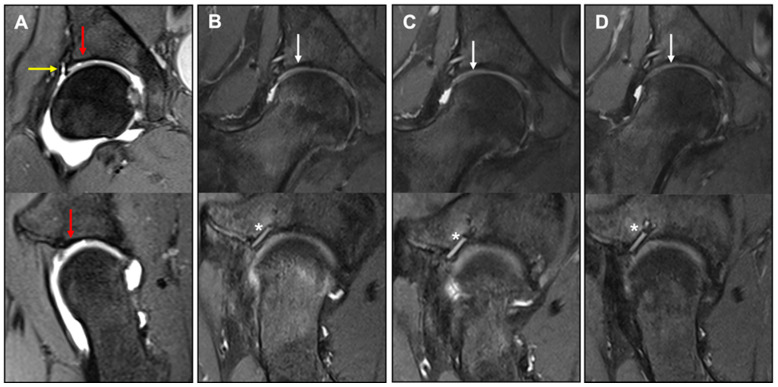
Coronar and sagittal preoperative arthro-MRI (A) with acetabular cartilage defect (red arrow) and labral tear (yellow arrow) and coronar and sagittal PD-TSE FS MRI 6 months (B), 12 months (C), and 24 months (D) postoperative with anker refixation of the labrum (asterisk) and cartilage repair tissue (white arrow) in the defect side with complete integration and a complete filling of the defect.
